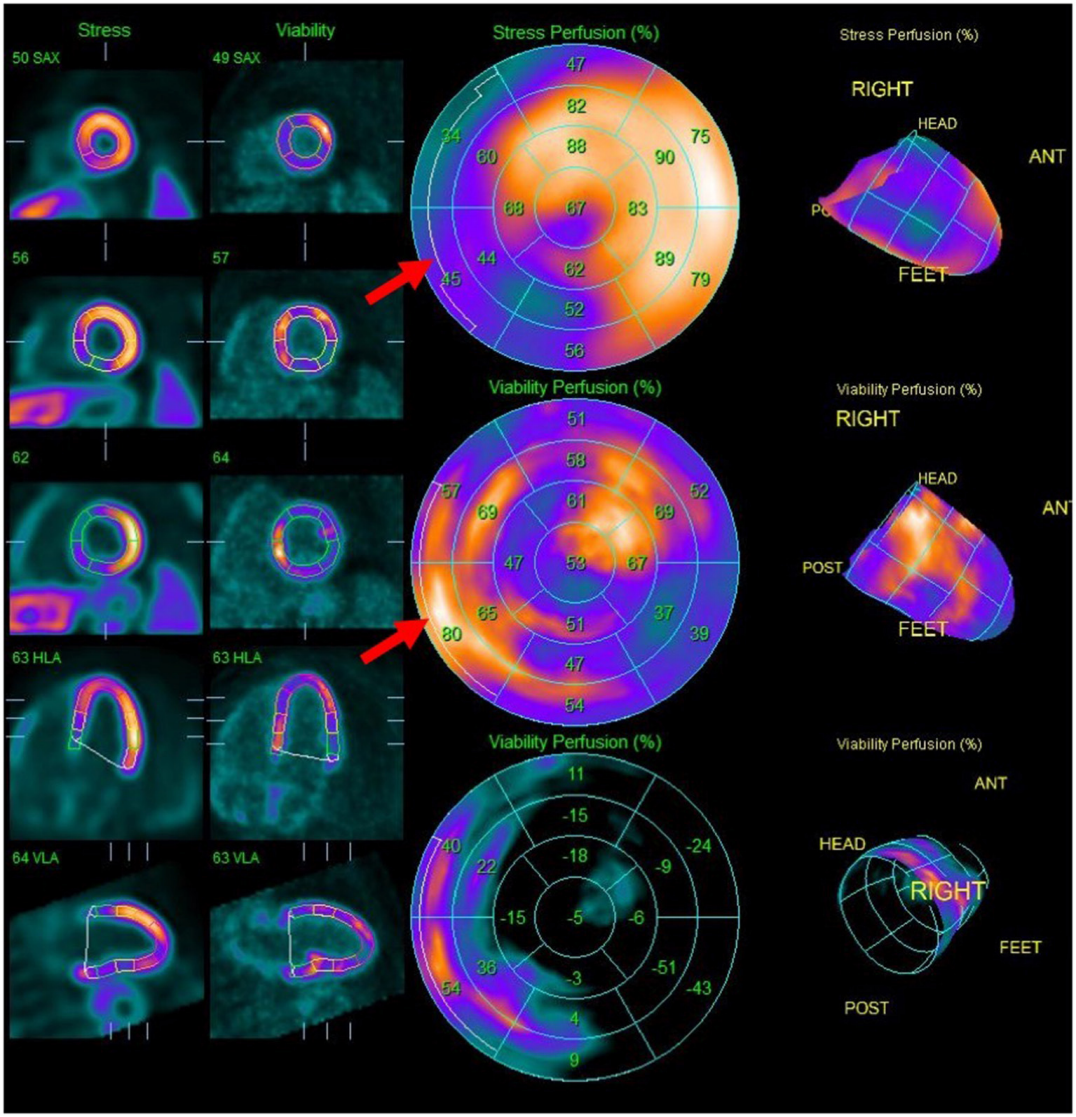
Splash images demonstrating moderate to severe transmural perfusion abnormality mainly involving the mid-base septal and inferoseptal wall (upper row), corresponding to areas of increased FDG uptake (middle row), denoting significant inflammation causing decreased perfusion (“mismatch” pattern). Note additional sites of increased FDG uptake without corresponding decreased perfusion such as in the mid-apical anterolateral wall.
